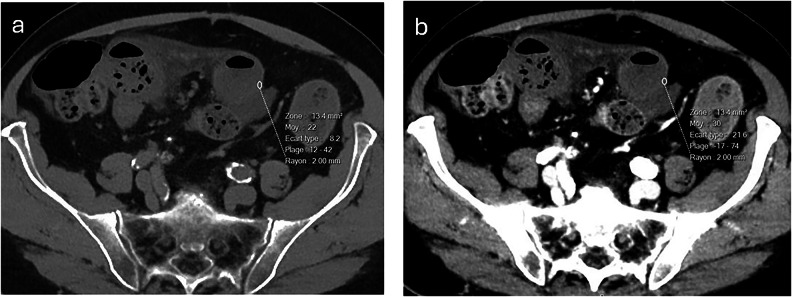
Axial 40 keV virtual monochromatic non-enhanced (a) and portovenous (b) multi-detector CT images demonstrate acute bowel ischaemia resulting from acute small bowel obstruction in an 86-year-old male patient. Quantitative analysis of the bowel wall revealed only minimal change in the mean bowel wall density during the portovenous phase (b), with a mean density of 30 Hounsfield units (HU) in b compared to 22 HU in a
